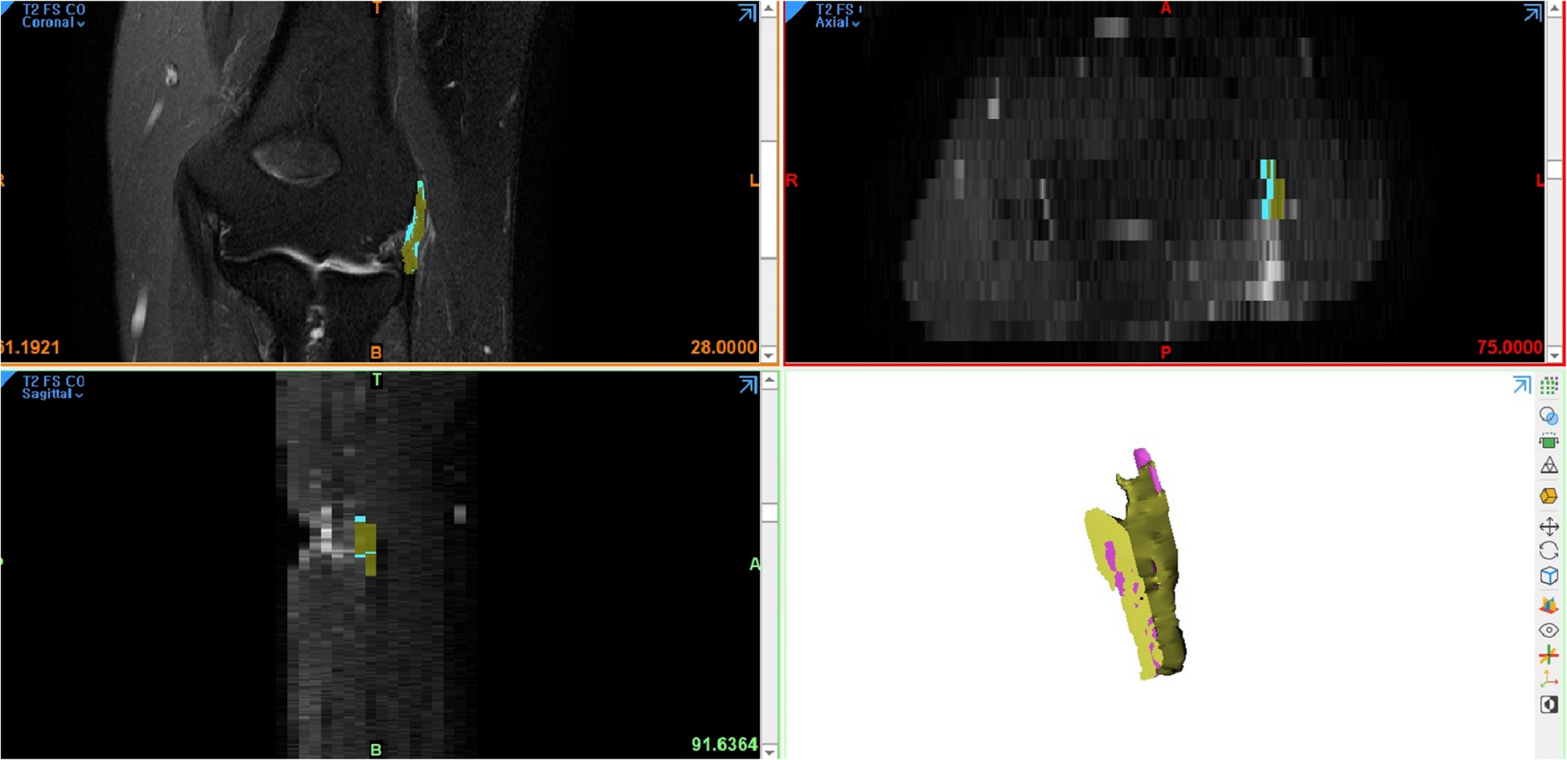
A screenshot of the three-dimensional (3D) graphic processing software. Digitized magnetic resonance imaging data in DICOM format were imported and the coronal, sagittal, and axial views of the computed tomography data were obtained in 3D graphic processing software (Mimics® 22.0, Materialise, Antwerp, Belgium)
